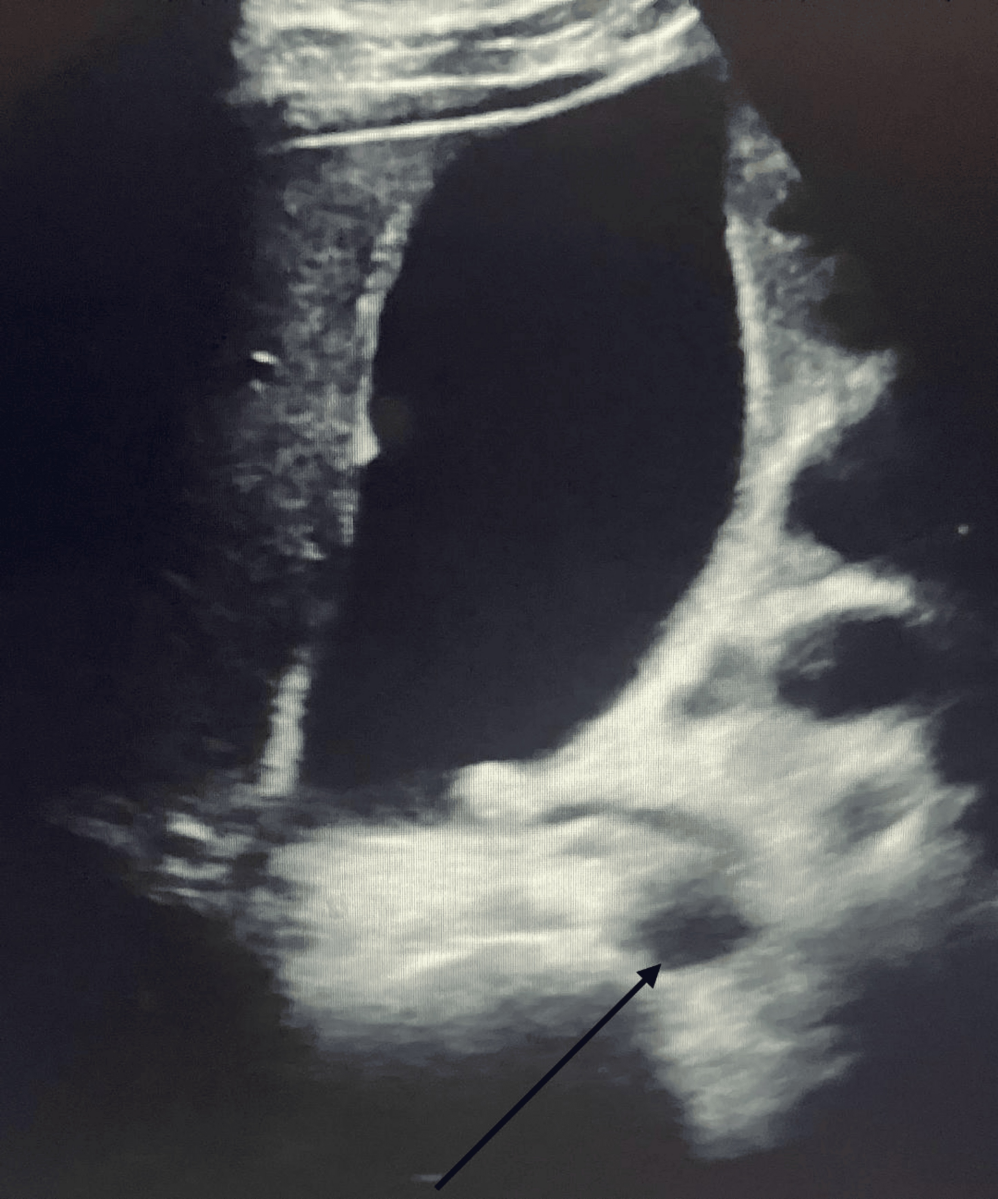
Ultrasound of the Abdomen The black arrow shows a dilated common bile duct measuring 5.8 mm. There are no stones in the gallbladder and no signs of acute cholecystitis.
